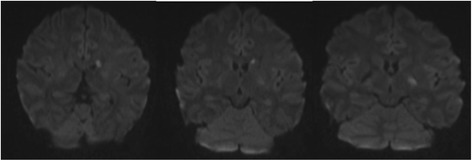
MRI images showing hyperintense lesions involving the left caudate nucleus and basal ganglia on DWI sequence due to restricted diffusion
